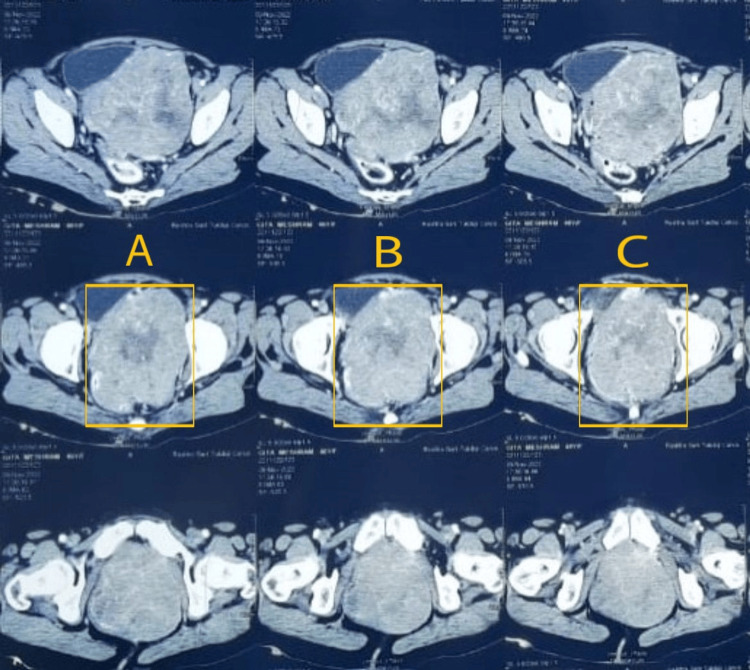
An enhanced MRI image showing a large, well-defined soft tissue mass with heterogenous enhancement in a bulky tumor The area highlighted in A-C shows the tumor MRI: magnetic resonance imaging
